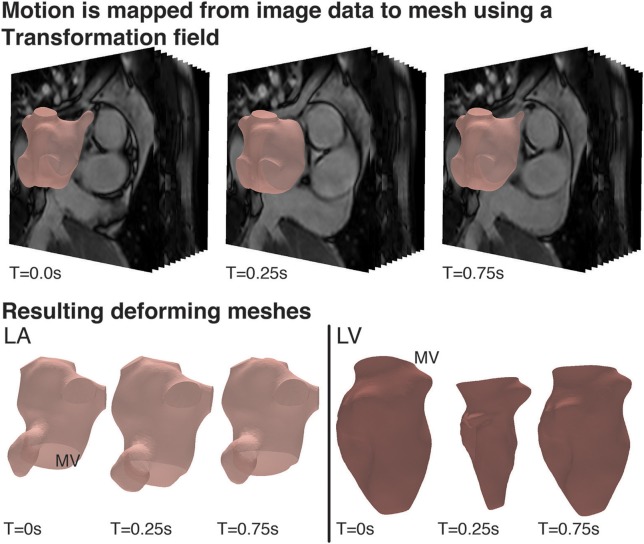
Cardiac Motion Tracking: (Top) Using the visualization and analysis package, Eidolon, an algorithm based on MIRTK which uses temporal-sparse free form deformations applied to the Cine MRI data to generate a transformation field for the entire image volume describing the motion of structures throughout the time-dependant image series, including the motion of the LA and LV. (Bottom) The tracking field was then applied to the LA and LV mesh and the deforming mesh was visually checked to ensure tracking was accurate throughout the cycle.
